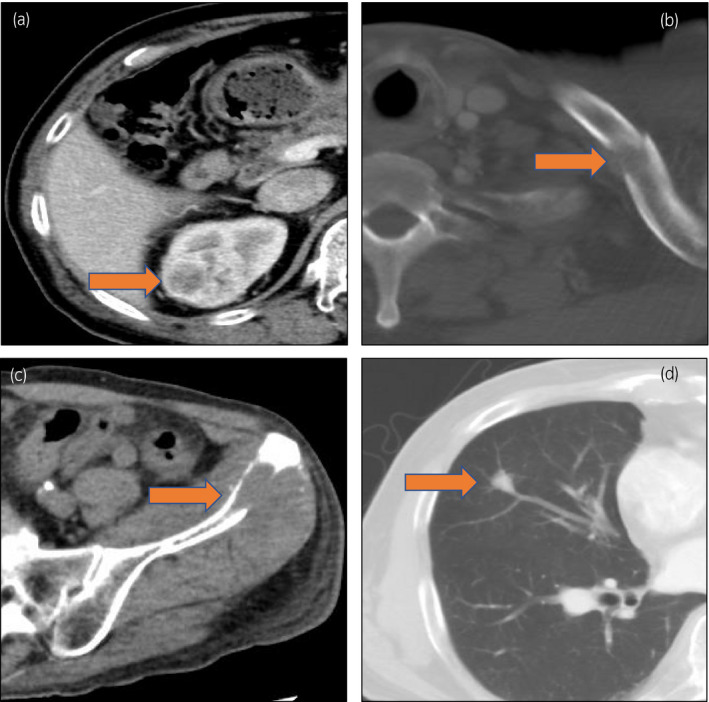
Abdominal and chest CT scan at the first diagnosis. Abdominal and chest CT shows the renal mass with early enhancement in right kidney with 35 mm diameter (a), osteolytic mass in the left clavicle (b) and left iliac crest (c), and small nodule in middle lobe of the right lung (d).
